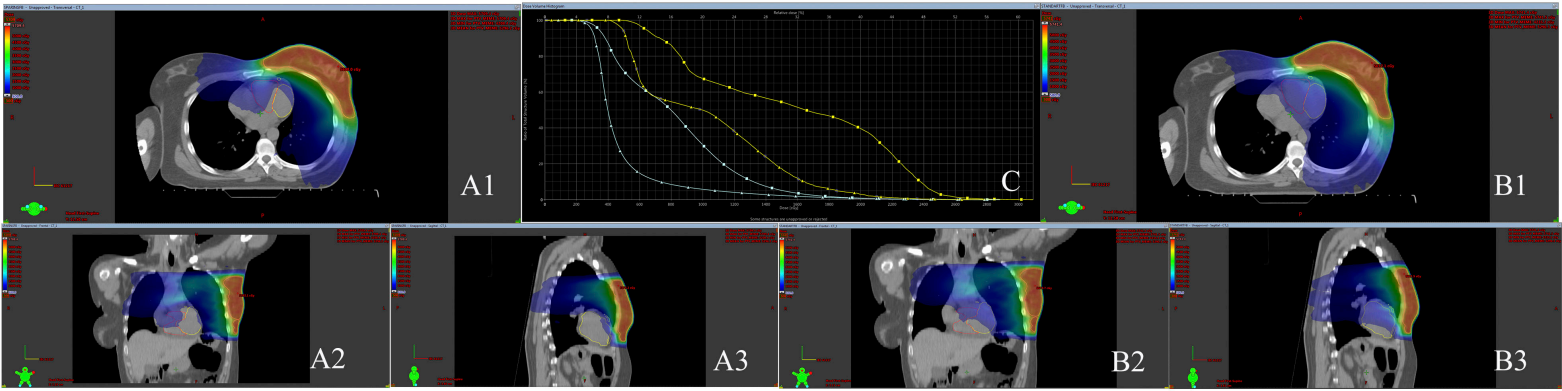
Cross-sectional CT images of a patient with the LAD and LV
